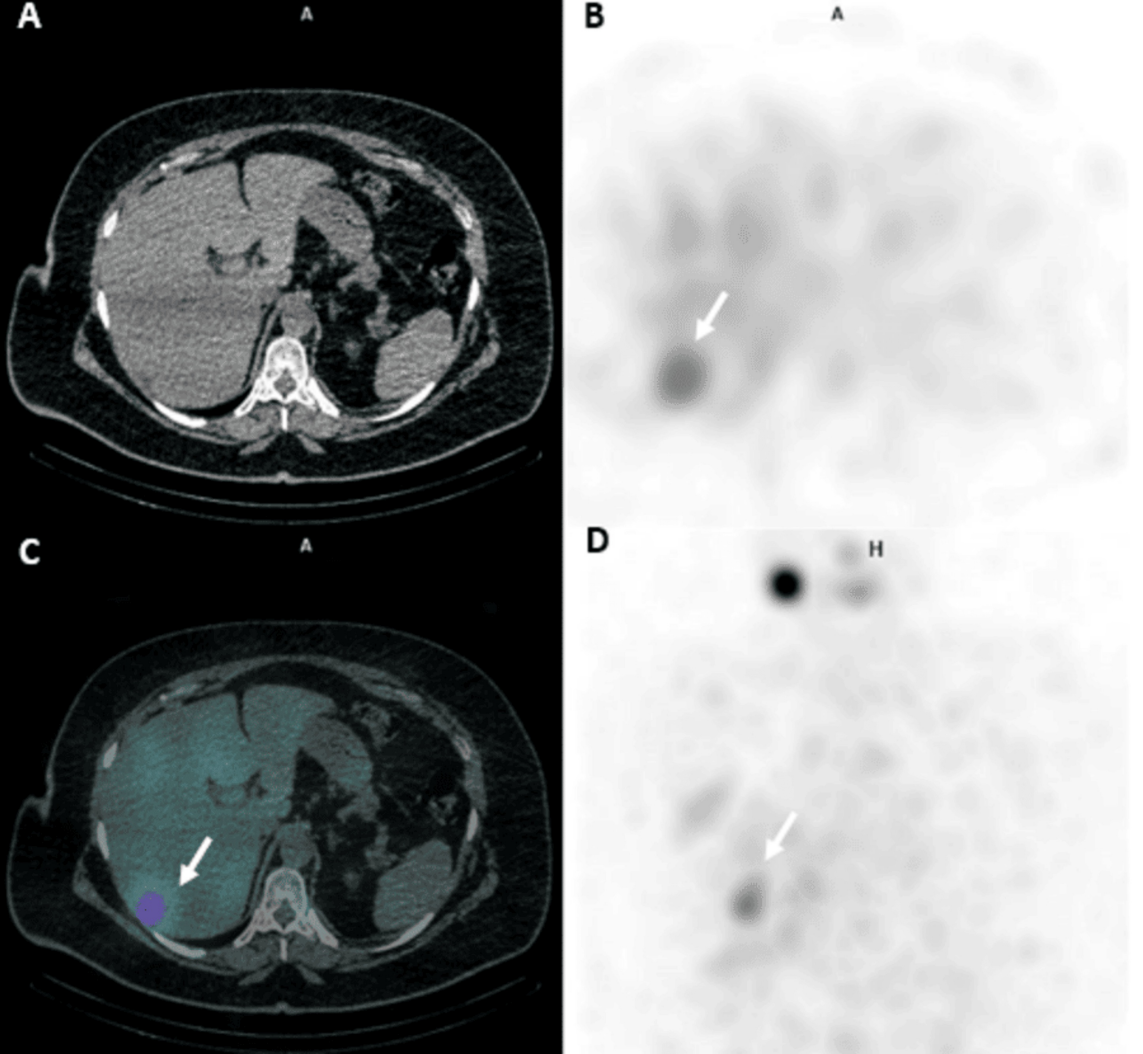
Post-ablation whole-body RAI SPECT/CT done (third RAI dose). Post-ablation whole-body RAI SPECT/CT done one week after the third RAI dose shows reappearance of the RAI uptake within hepatic lesion in segment VII (white arrow) demonstrated on SPECT, fused, and MIP images (B, C, and D respectively). RAI, radioactive iodine; SPECT/CT, single photon emission computed tomography/computed tomography; MIP, maximum intensity projection
